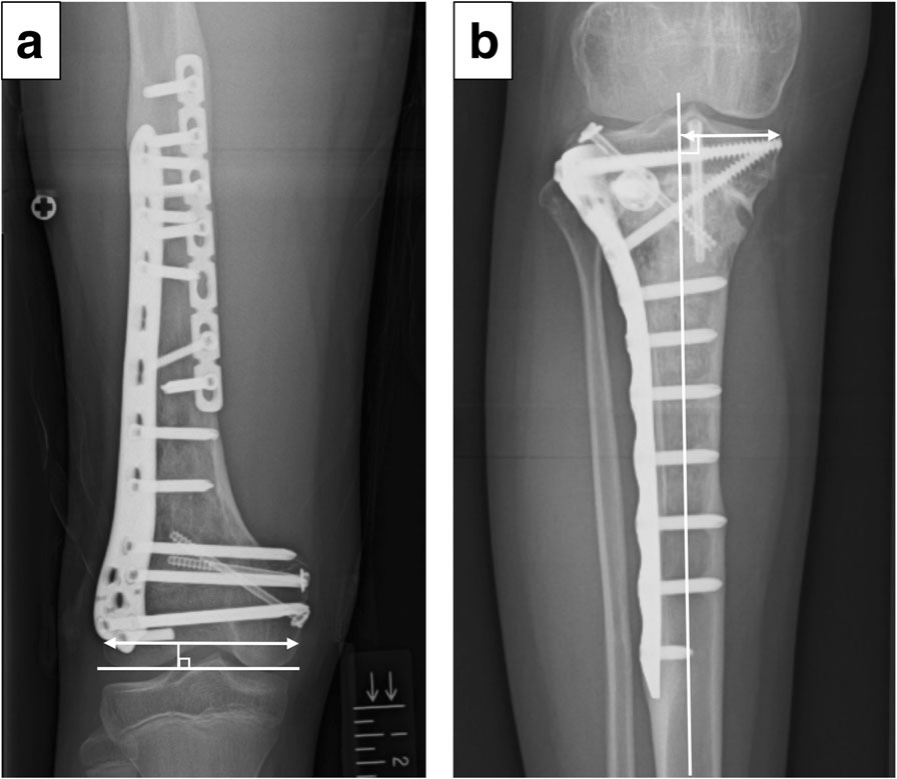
The width of epiphysis was measured by using whole standing leg radiographs with a 1-mm precision scale. The distance between the intersection of the line that was placed parallel to the joint surface and passed through the medial and lateral cortex at the level of intercondylar fossa in the distal femur before surgery and at the latest examination (a 10-year-old boy with osteosarcoma of the right distal femur, 55 months after surgery) (a). The width of the epiphysis of the proximal tibia was measured by a line that was perpendicular to the tibial anatomical axis and passes through the medial edge of the medial condyle. The distance between the ends of the medial and the cross point to the mechanical axis was measured on the proximal tibia before surgery and at the latest examination (an 11-year-old girl with osteosarcoma of the right proximal tibia, 42 months after surgery) (b)
